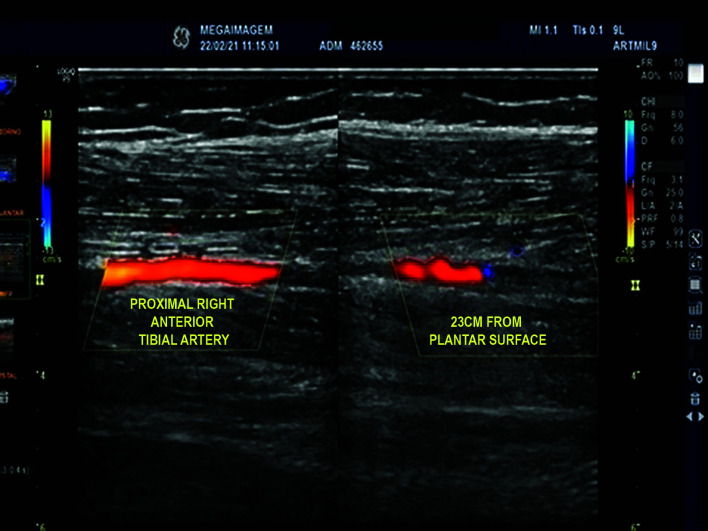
Arterial Doppler image showing occlusion of the right anterior tibial artery at 23 cm from the plantar surface.
